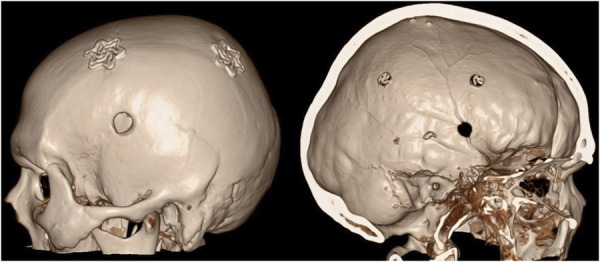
Left, A left-lateral 3D CT reconstruction of patient 4 demonstrating the position of the navigated pterional burr hole slightly posterior compared to Figure 3, here without a cover plate. Right, A view from the inside-out perspective, displaying the inner skull surface, demonstrating projection of the pterional burr hole on the MMA course. 3D, 3-dimensional; CT, computed tomography; MMA, middle meningeal artery.
